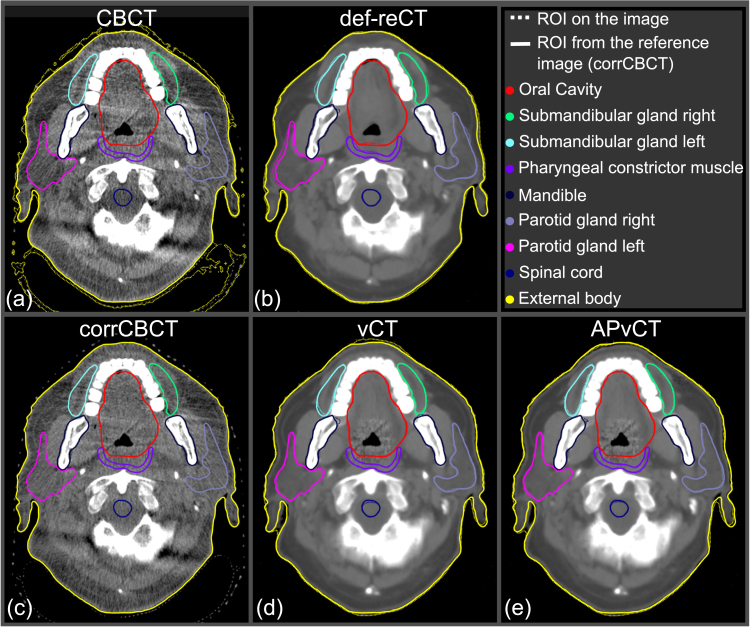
An axial CT slice from (a) cone beam computed tomography (CBCT), (b) deformed repeat CT (def-reCT), (c) corrected CBCT (corrCBCT), (d) virtual CT (vCT), and (e) anatomy-preserving virtual CT (APvCT). Separate deformable image registrations with mutual information as a similarity measure were created between planning CT and synthetic CTs/def-reCT to map the regions of interest (ROIs) to the image sets (with dotted lines). ROIs with solid lines denote the contours of reference daily anatomy from the corrCBCT.
